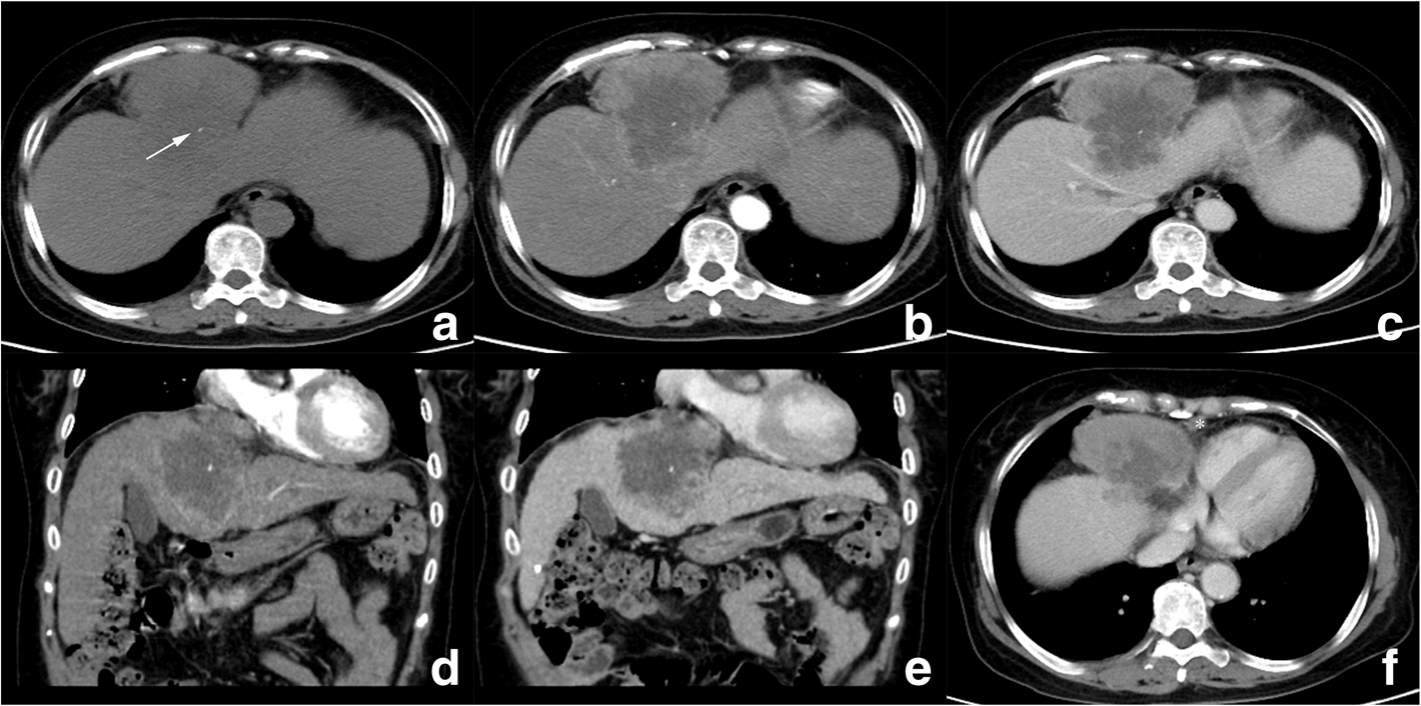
Computed tomography (CT) images of patient 2. a Unenhanced CT images reveal a hypodense mass in segment 4 with unclear margin and spot calcification (arrow). Tumor showed significant enhancement in peripheral portion in AP (b) and washout in PP (c); d and e are coronal reconstructed PP images, present large central part of cystic degeneration in the tumor; right phrenic angle lymphadenopathy is also observed (f, *)
